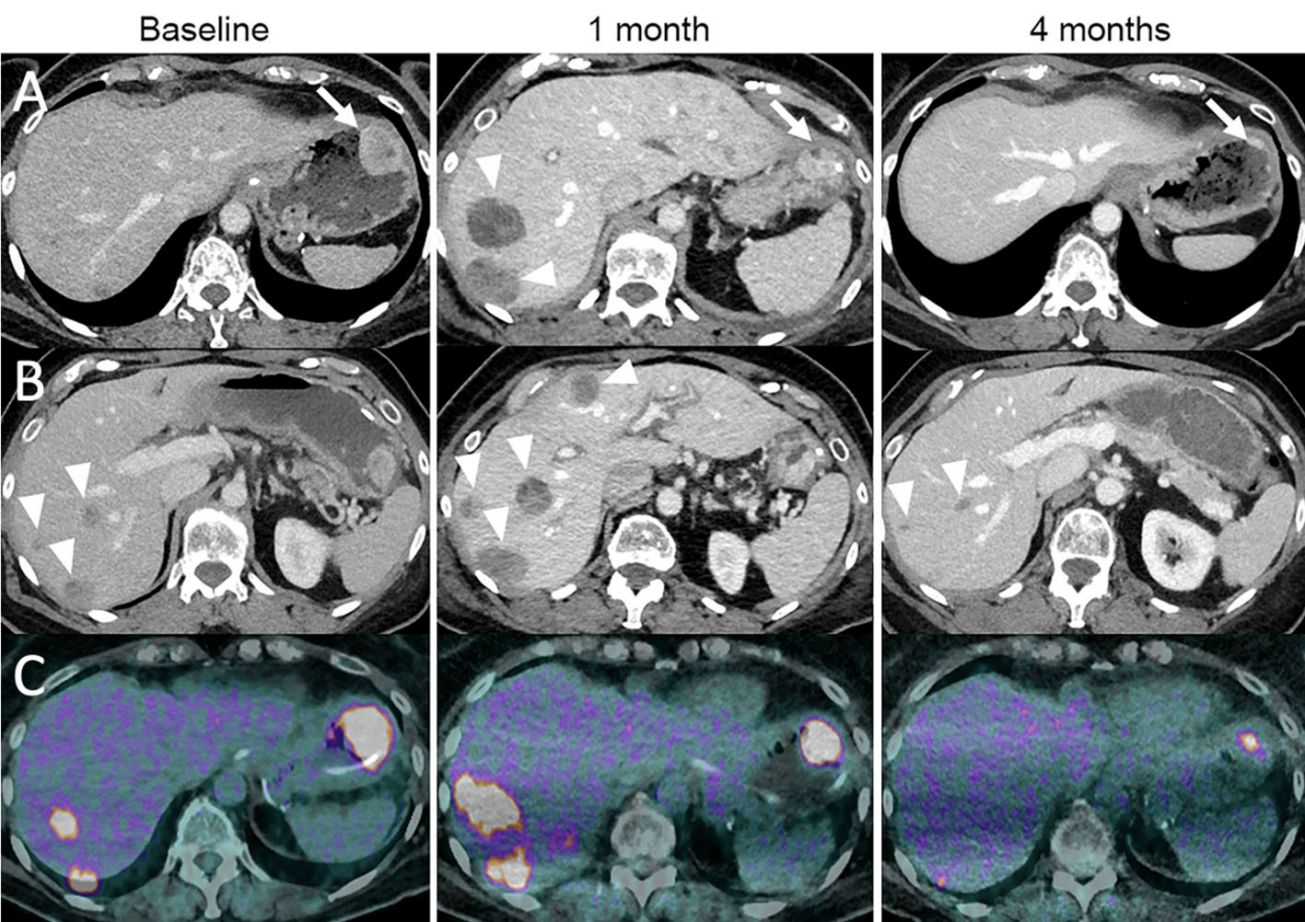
Dissociated response in a 68-year-old female with cutaneous melanoma of the back who progressed following ipilimumab (anti-CTLA-4) and nivolumab (anti-PD-1). The patient was given tumor-infiltrating lymphocyte-adoptive cell therapy (TIL-ACT). Baseline contrast-enhanced CT at the portal venous phase (A, B) and ^18^F-FDG-PET/CT (C) show a gastric wall metastasis (arrow) and liver metastases (arrowheads). 1-month follow-up imaging after TIL-ACT demonstrates a decrease in the gastric wall metastasis (arrow). However, liver metastases increased in size (arrowheads). 4-month follow-up imaging shows a marked decrease in the gastric wall metastasis (arrow) and liver metastases (arrowheads).
